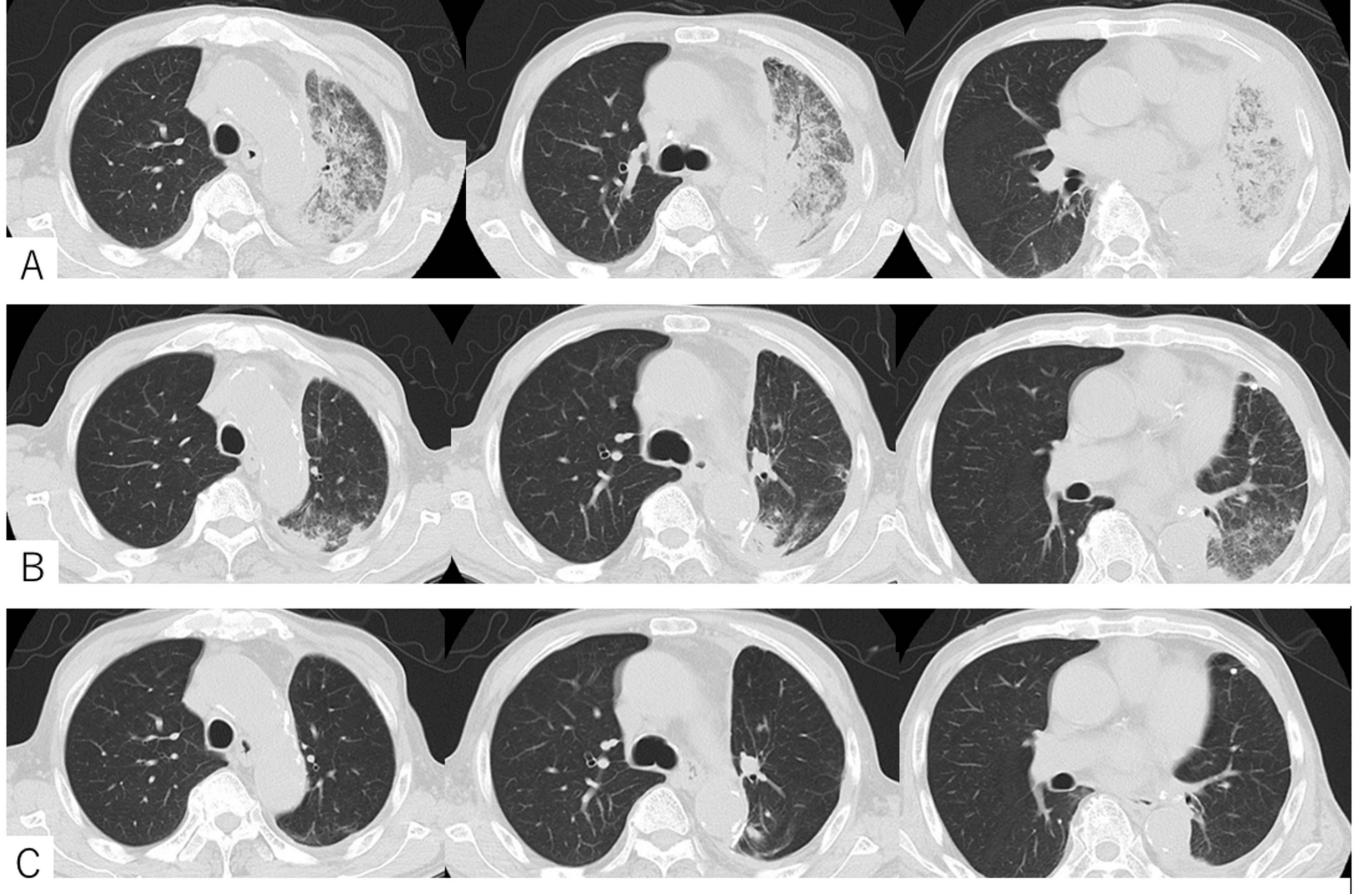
Clinical course after treatment with a BRAF inhibitor A:  Chest computed tomography at the start of treatment. B:  Five weeks after treatment. C:  Status at three months after treatment was maintained until six months after treatment.
